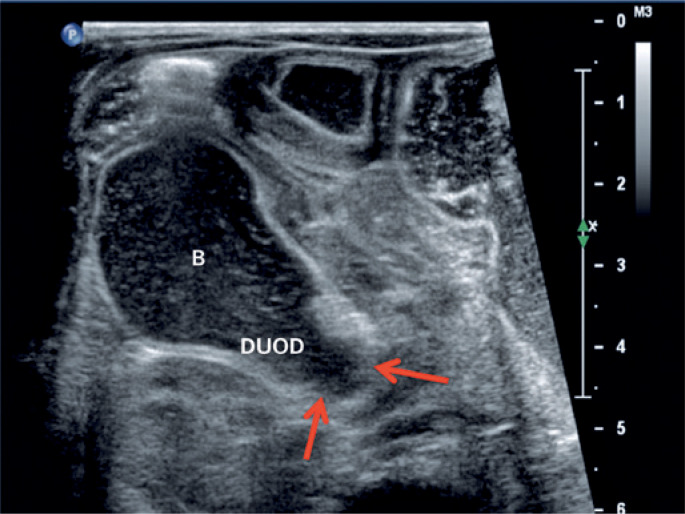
Ultrasonography with a high frequency linear transducer shows distension of the duodenal bulb, (B) with abrupt tapering in the second duodenal portion, corresponding to the local duodenal membrane
